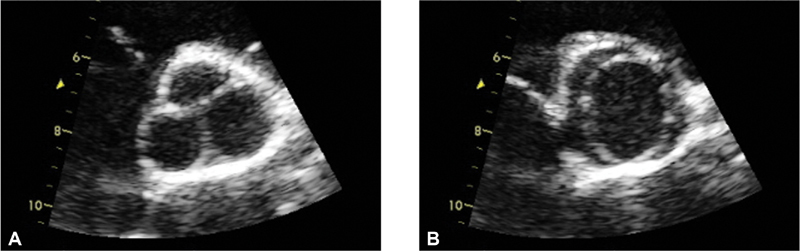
Transthoracic echocardiogram of bicuspid aortic valve (BAV), short axis view. (
